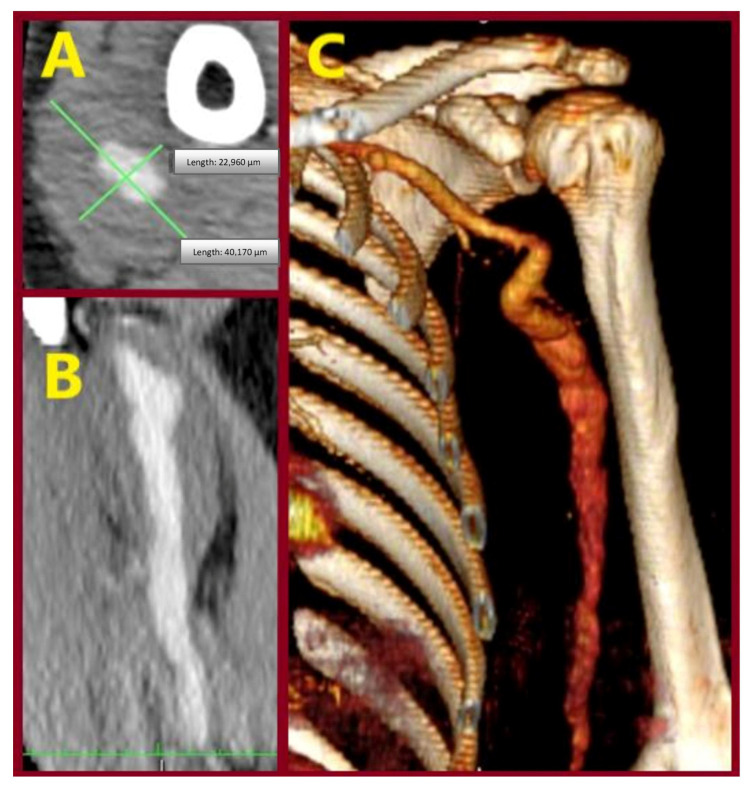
Computed tomography angiography depicting the brachial aneurysm. (A) Axial image, (B) coronal image, (C) 3-D reconstruction.
